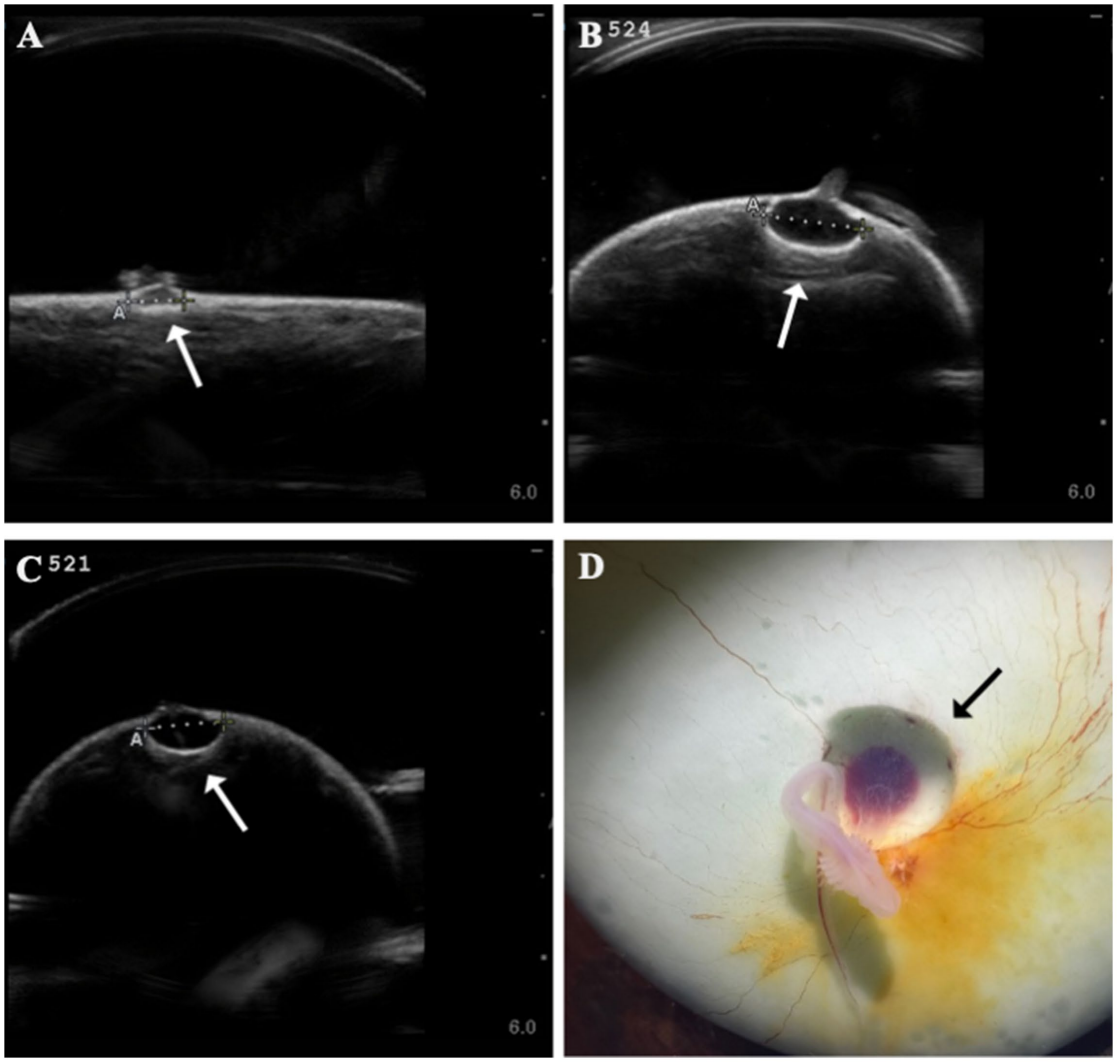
Formation of anechoic fluid-filled vesicles (arrows) at the base of the yolk stalk as seen on ultrasound in zebra shark (*Stegostoma tigrinum*) eggs (A–C). The vesicle was documented to expand in the same egg (#524) from 44 days post-oviposition (A) to 79 days post-oviposition (B). Vesicles were confirmed at necropsy (D).
